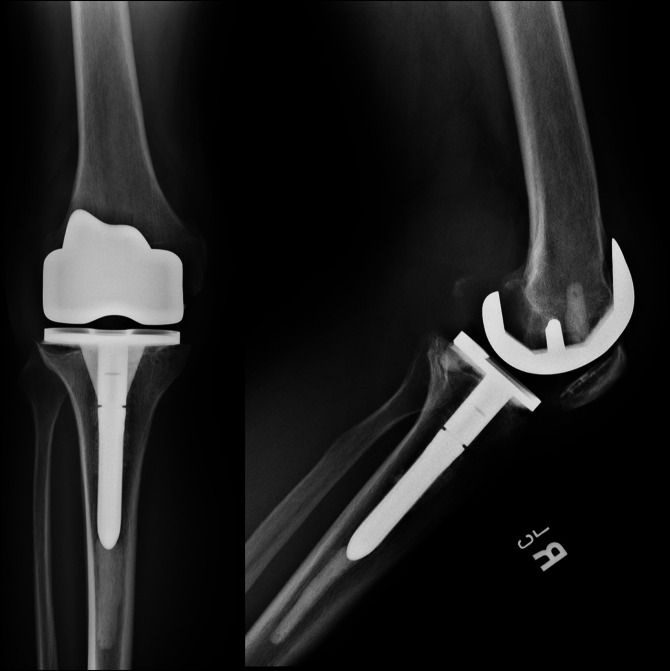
AP (left) and lateral (right) radiographs showing the right knee 2 years after the initial total knee arthroplasty and 2 months before one-stage revision. Extensive osteolysis is seen around the femoral and tibial implants.
